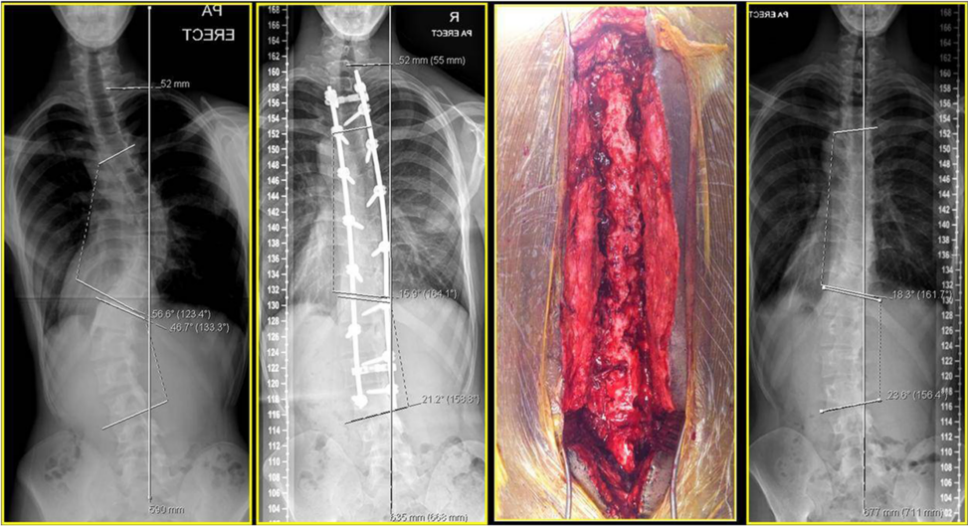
Late deep MRSA infection in a 15/♂ showing pre-op and 47 months post-op x-rays (i.e., immediately prior to implant removal). Solid arthrodesis was seen at implant removal and final x-rays 6 months post implant removal are depicted
